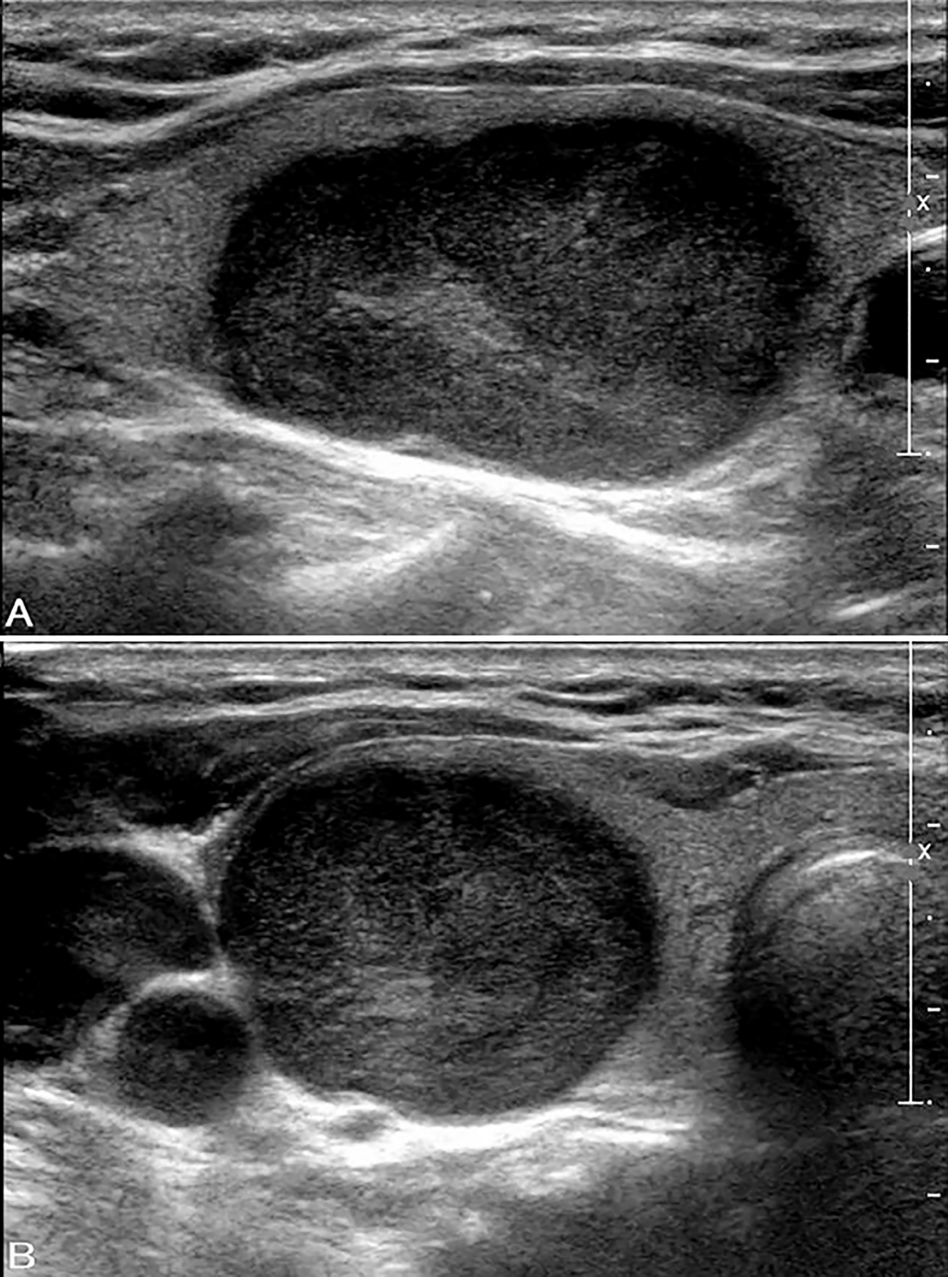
Ultrasound images in a patient with FTC in the right lobe. A, B. Longitudinal (A) and transverse (B) US show a very hypoechoic solid thyroid nodule (size: 32 mm × 19 mm × 24 mm) with wider-than-tall shape and smooth margins. The nodule was classified as ACR TIRADS category TR4. The cytological diagnosis was follicular neoplasm with negative *K-RAS* mutation.
